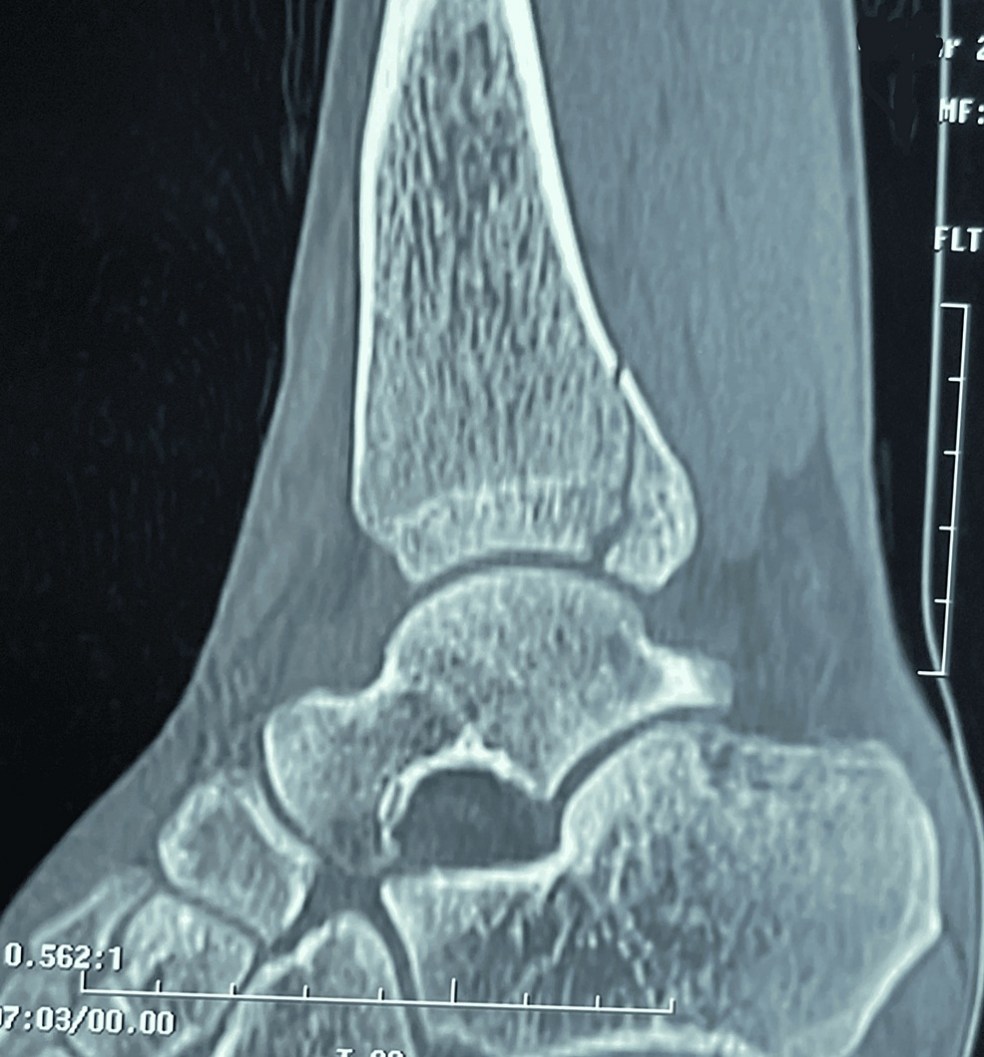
Sagittal section of the non-enhanced CT scan of the ankle confirming the isolated fracture of the posterior margin
